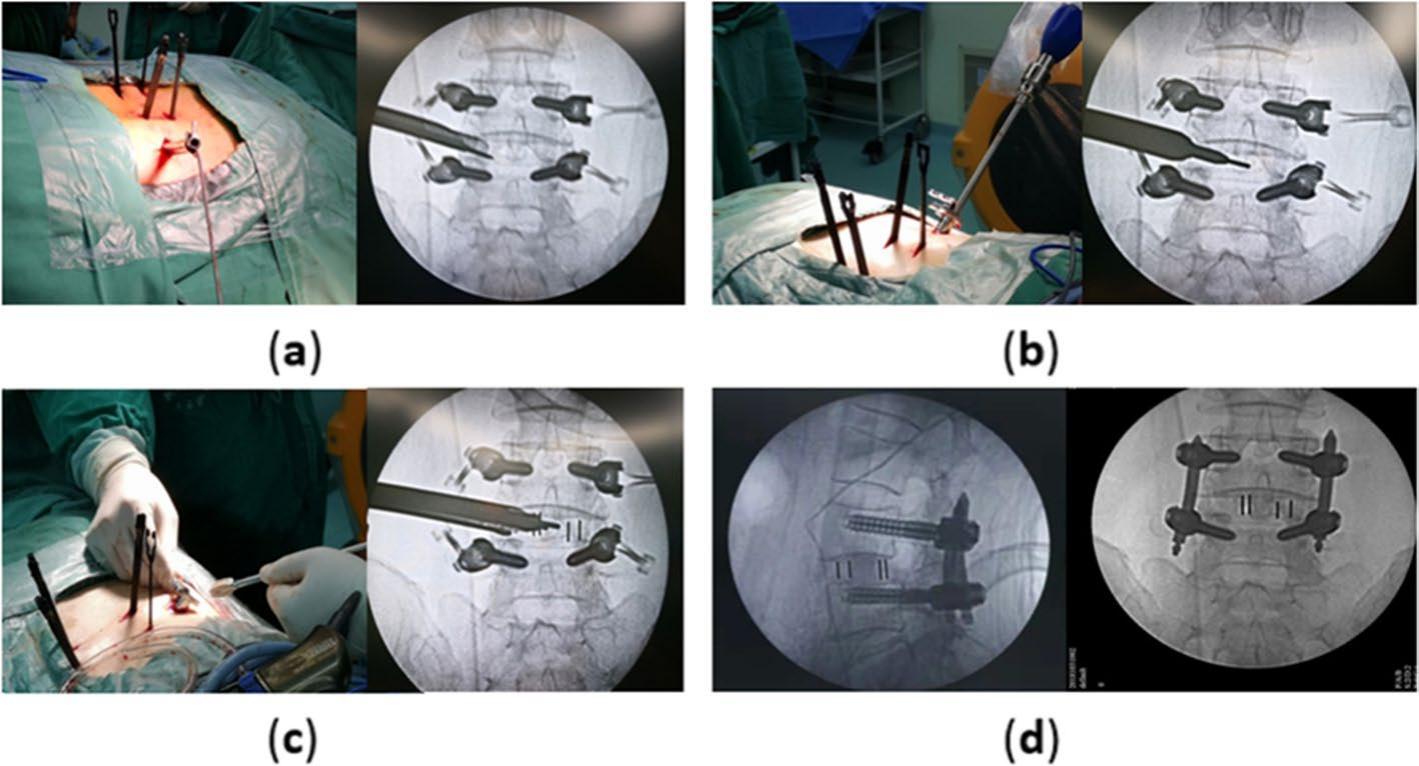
a Bilateral percutaneous pedicle screw instrumentation and the working tube placement; b Lumbar discectomy was performed under endoscopic spinal system; C A cage was implanted via a tail-end expandable tubular system; d Postoperative plain films of lumbar spine
